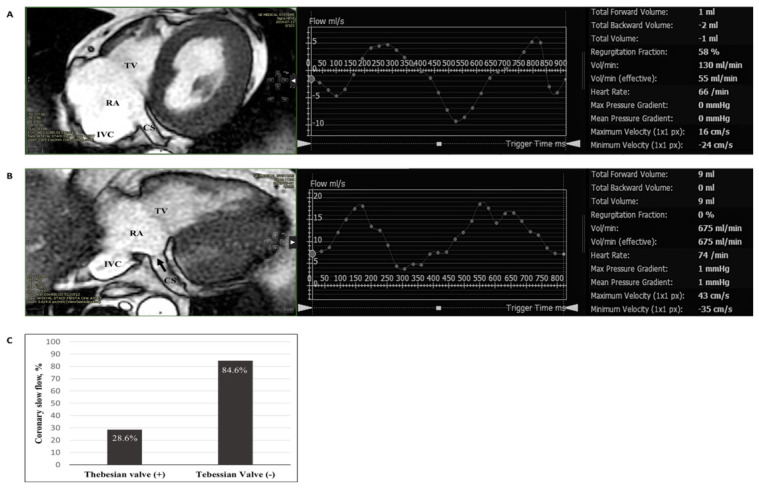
Cardiac magnetic resonance imaging. (A) In a patient without a Thebesian valve in the CS, coronary venous regurgitant flow is reflected in the flow–time curve on the left; (B) in a patient with a Thebesian valve in the CS (black arrow), the flow–time curve shows anterograde flow, with no regurgitant flow detected; (C) only 28.6% of patients with a Thebesian valve in the CS have slow coronary flow. Abbreviations: coronary sinus (CS), inferior vena cava (IVC), right atrium (RA), tricuspid valve (TV). Adapted from our previous study [52].
